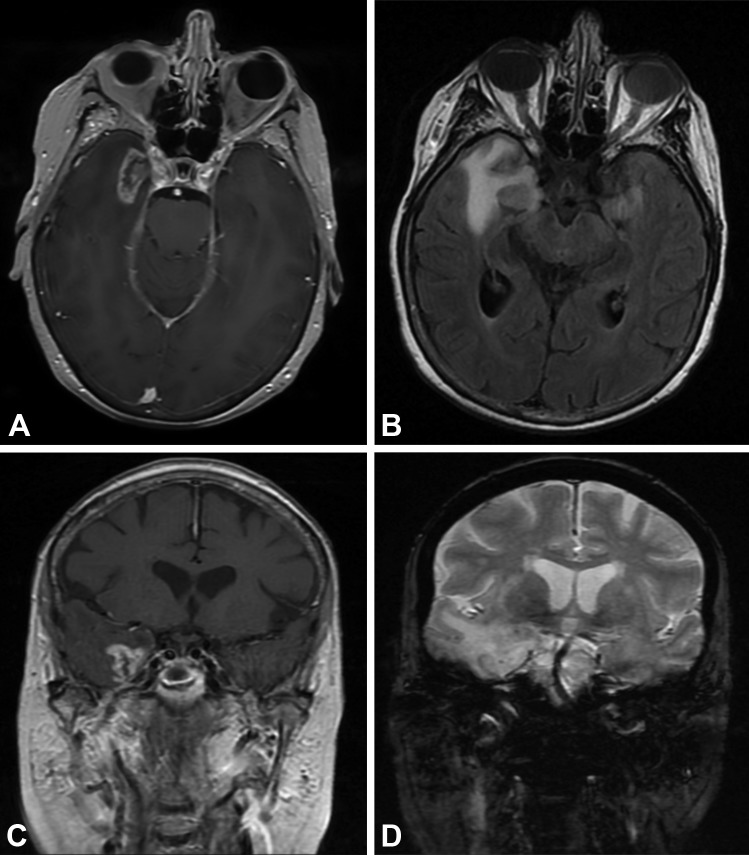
Radiation Necrosis Magnetic resonance images at presentation of a 73-year-old female with a history of trigeminal neuralgia, which was treated previously by TomoTherapy radiosurgery, shows significant mass effect in close proximity to Meckel’s cave. (A) Axial T1-weighted image with contrast; (B) axial FLAIR; (C) coronal T1-weighted image with contrast; and (D) coronal T2-weighted image. *Used with permission from Barrow Neurological Institute.*
